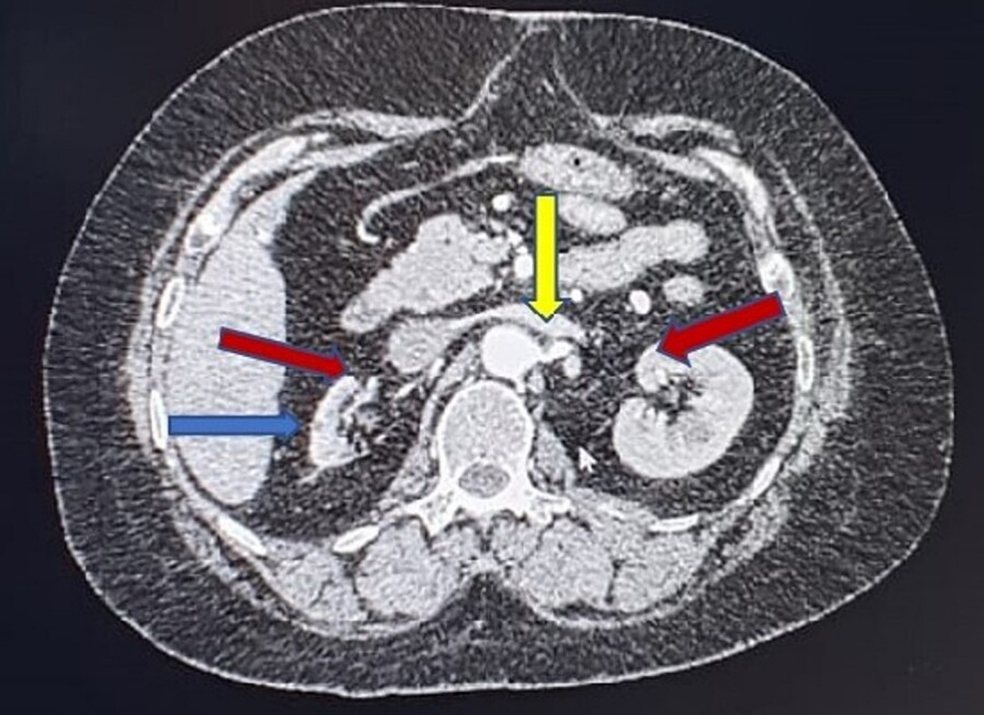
CT angiogram abdomen (axial view). Blue arrow indicates atrophic right kidney. Red arrows indicates bilateral renal artery stenosis. Yellow arrow indicates stenosis of inferior mesenteric artery origin.
